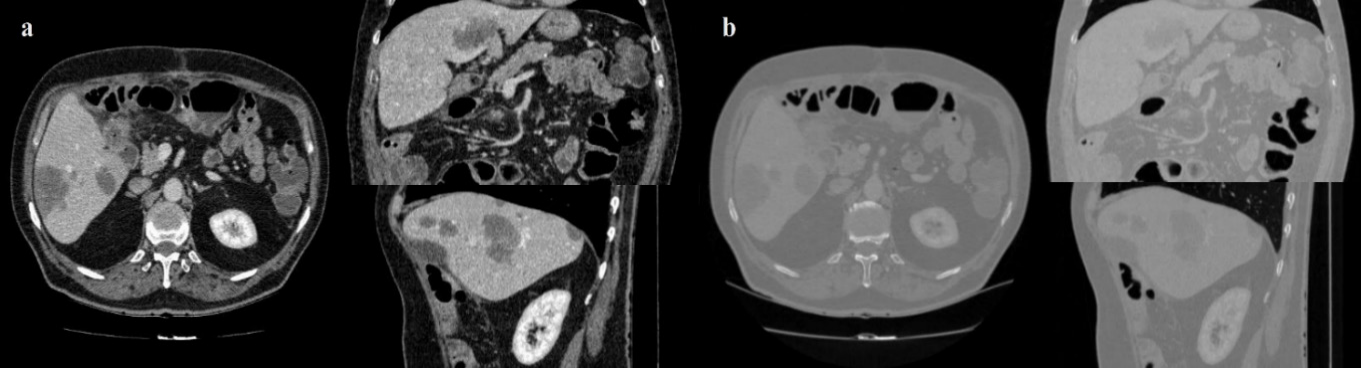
CT image with window level and settings recommendations for Abdominal CT (a), CT image with dynamic window level and settings (b)
